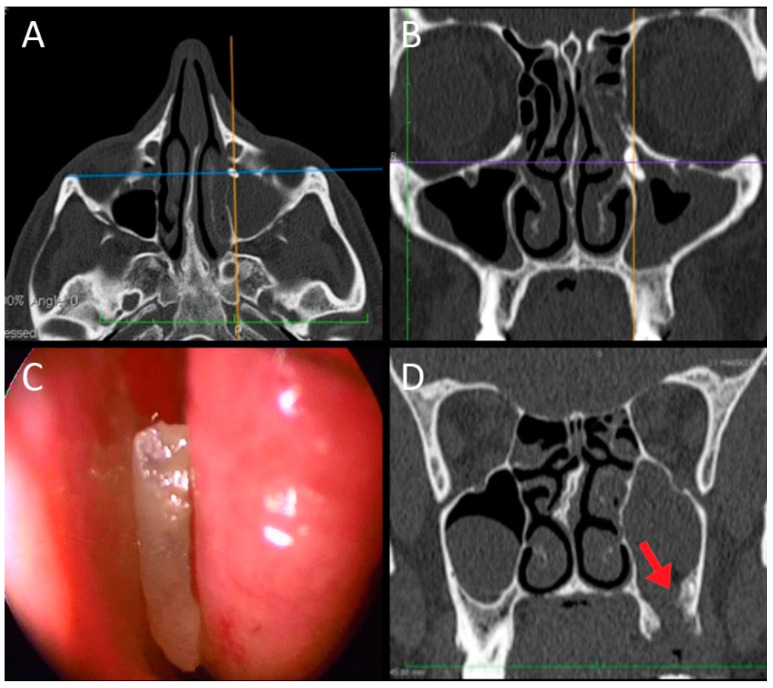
Male patient, 61 years old, complaining of sinus pain, nasal obstruction, and rhinorrhea. CT ((A): axial scan; (B): coronal scan) revealed the presence of a foreign body in the left osteomeatal complex. Endoscopic surgery was performed to remove the foreign body (C) and to clean the maxillary sinus. Because of wide oroantral fistula (D), red arrow surgical closure using a local mucosal flap was required.
